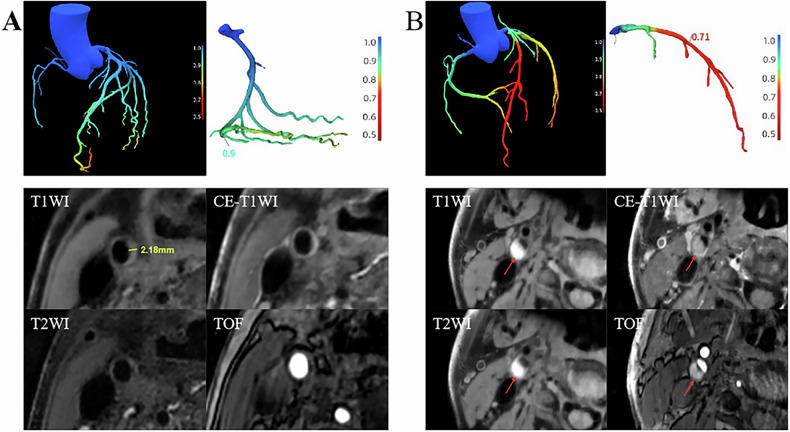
Carotid plaque imaging features in patients stratified by the presence of functional coronary artery stenosis. A A patient without coronary functional stenosis, whose lowest CT-FFR value is 0.90. The carotid artery wall thickness is 2.18 mm, which is less than 3 mm, and there are no features of complicated plaque or ulcerations. As a result, the patient’s Carotid Plaque-RADS is classified as level 2. B A patient with coronary functional stenosis, whose lowest CT-FFR value is 0.71. Intraplaque hemorrhage (indicated by arrows) is observed in the right internal carotid artery, and the patient’s Carotid Plaque-RADS is classified as level 4. T1WI, T1-weighted imaging; CE-T1WI, contrast-enhanced T1-weighted imaging; T2WI, T2-weighted imaging; TOF, time-of-flight imaging
